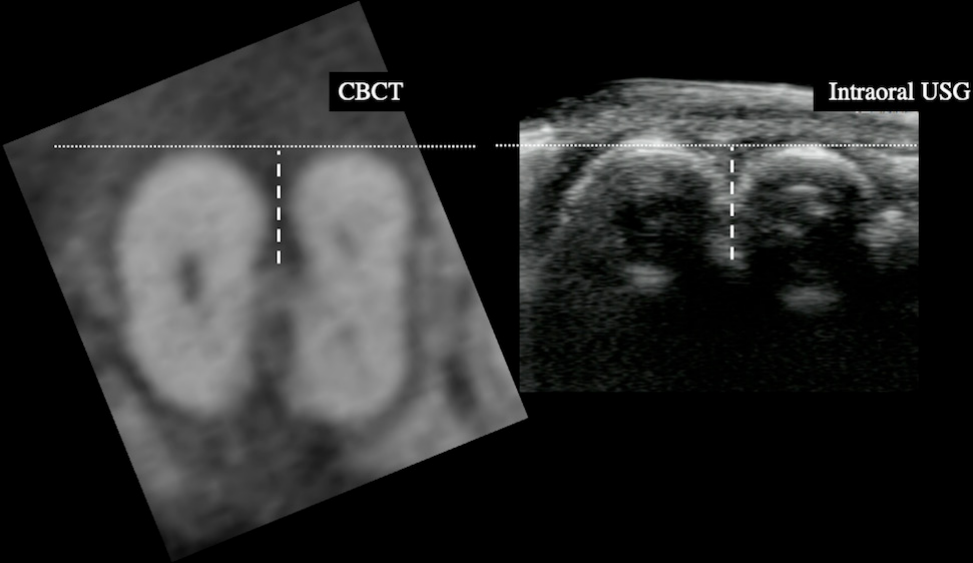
Measurement of the horizontal depth of furcation involvement on CBCT and intraoral USG images. Measurement was performed from the buccal surface of the roots to the end of the bone defect. The line with larger dots shows the measurement. CBCT, cone beam CT; USG, ultrasonography.
